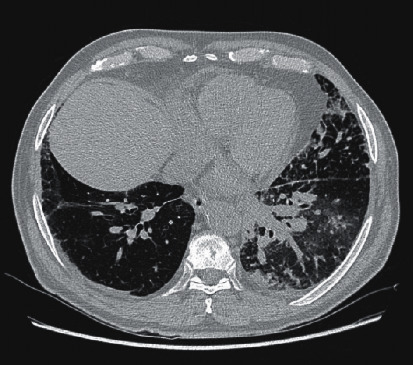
Computed tomography of chest showing transverse view of lungs with multiple patchy ground glass opacities with predominant lower lobe involvement, more than a month after initial presentation.
